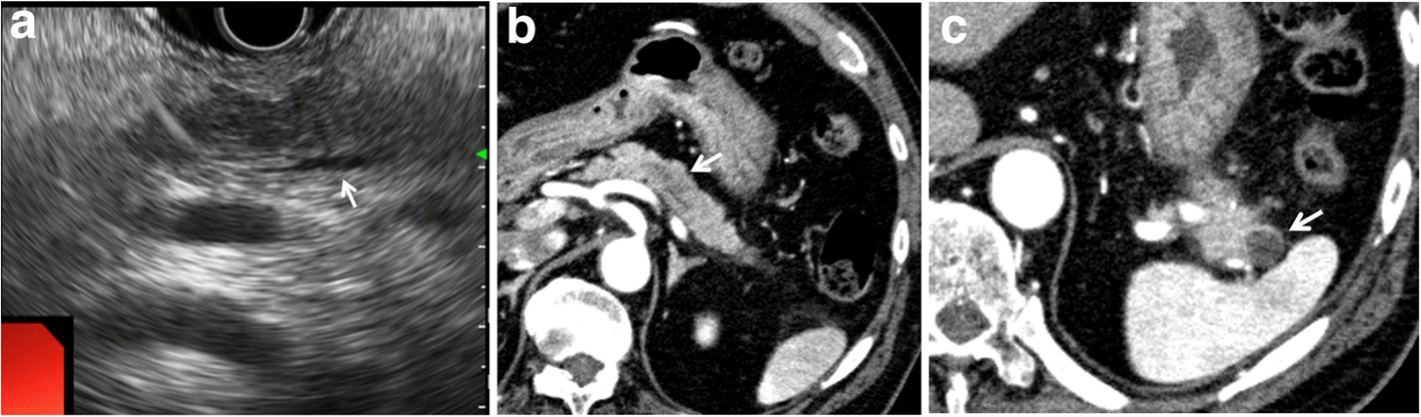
a Endoscopic ultrasonography showed a diffuse low-echoic area at the pancreatic body (Pb) and a slightly dilated upstream main pancreatic duct (MPD) (white arrow). b Enhanced computed tomography (CT) demonstrated an ill-defined, low-density area at the Pb (white arrow). c In the pancreatic tale (Pt), CT revealed a well-demarcated, low-density lesion with faint marginal enhancement
